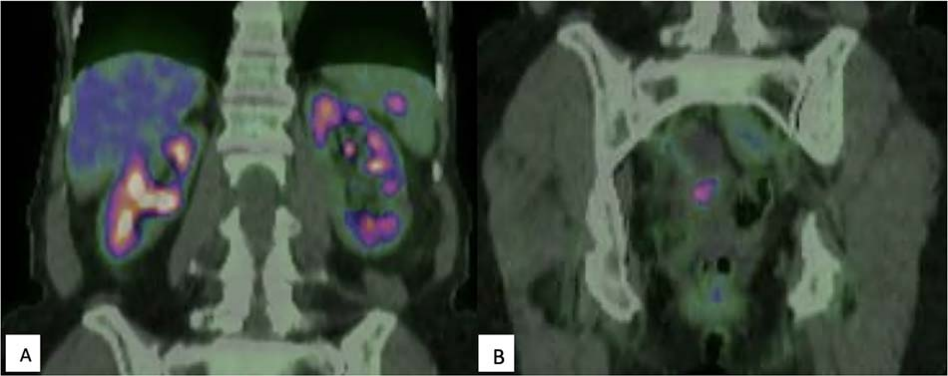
(A) FDG-PET demonstrating moderately intense (SUVmax 5.6) uptake within the spleen, suspicious for malignancy; (B) Multicystic pelvic lesion with solid component (SUVmax 4.0).
